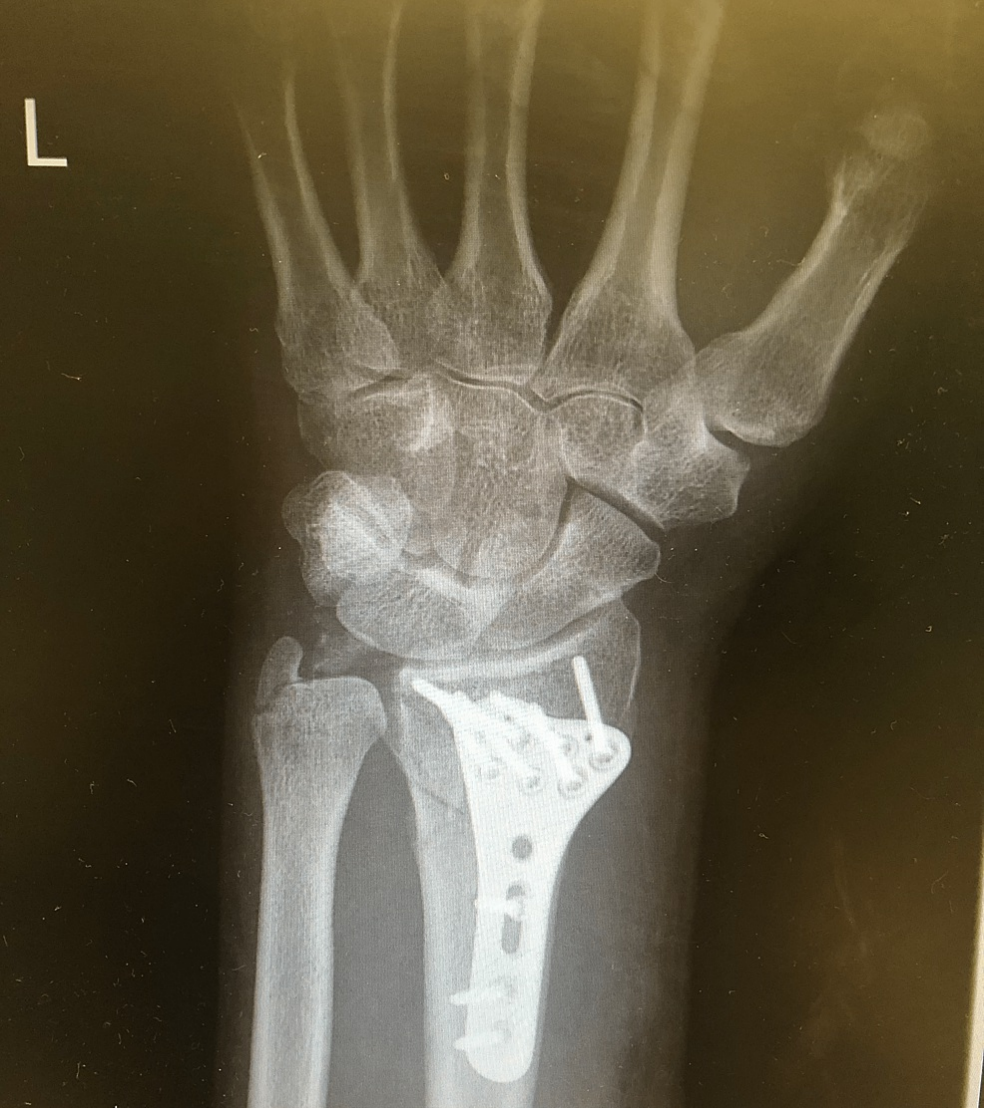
X-ray done after open reduction and internal fixation.
